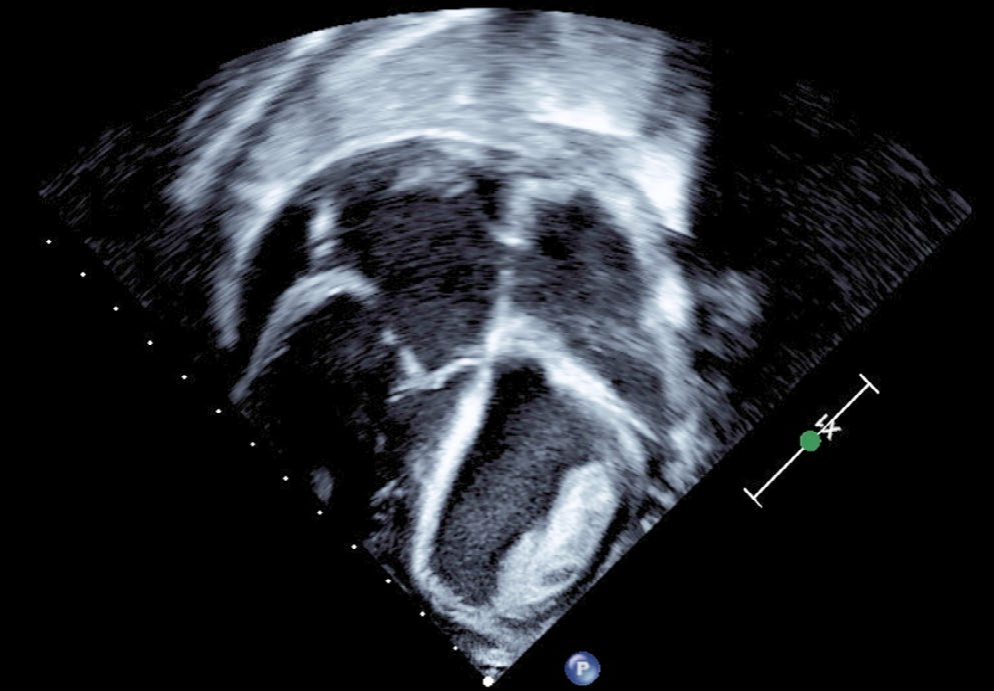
Echocardiography of Case 5 showing large echogenic mass attached to the anterior left ventricular wall
